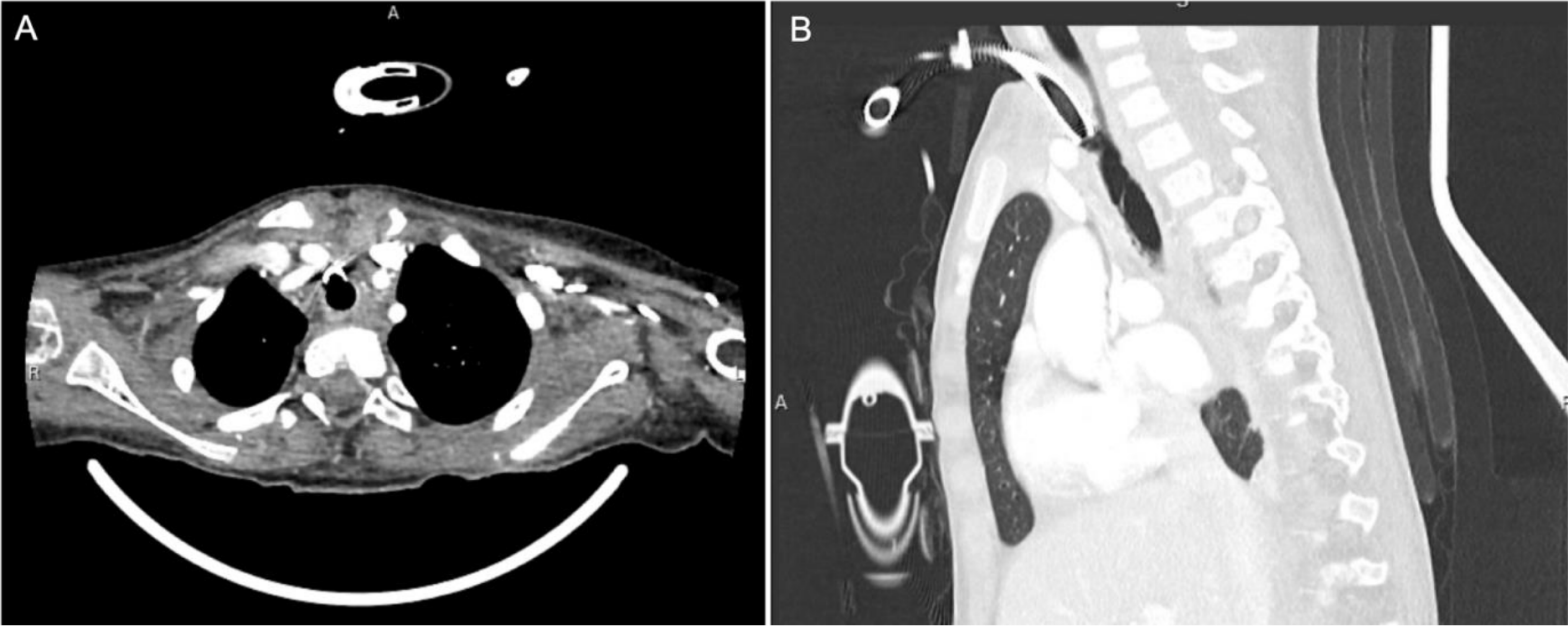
CT chest with contrast showing the close proximity between the tip of the tracheostomy tube and the innominate artery. (A) Axial plane. (B) Sagittal plane.
